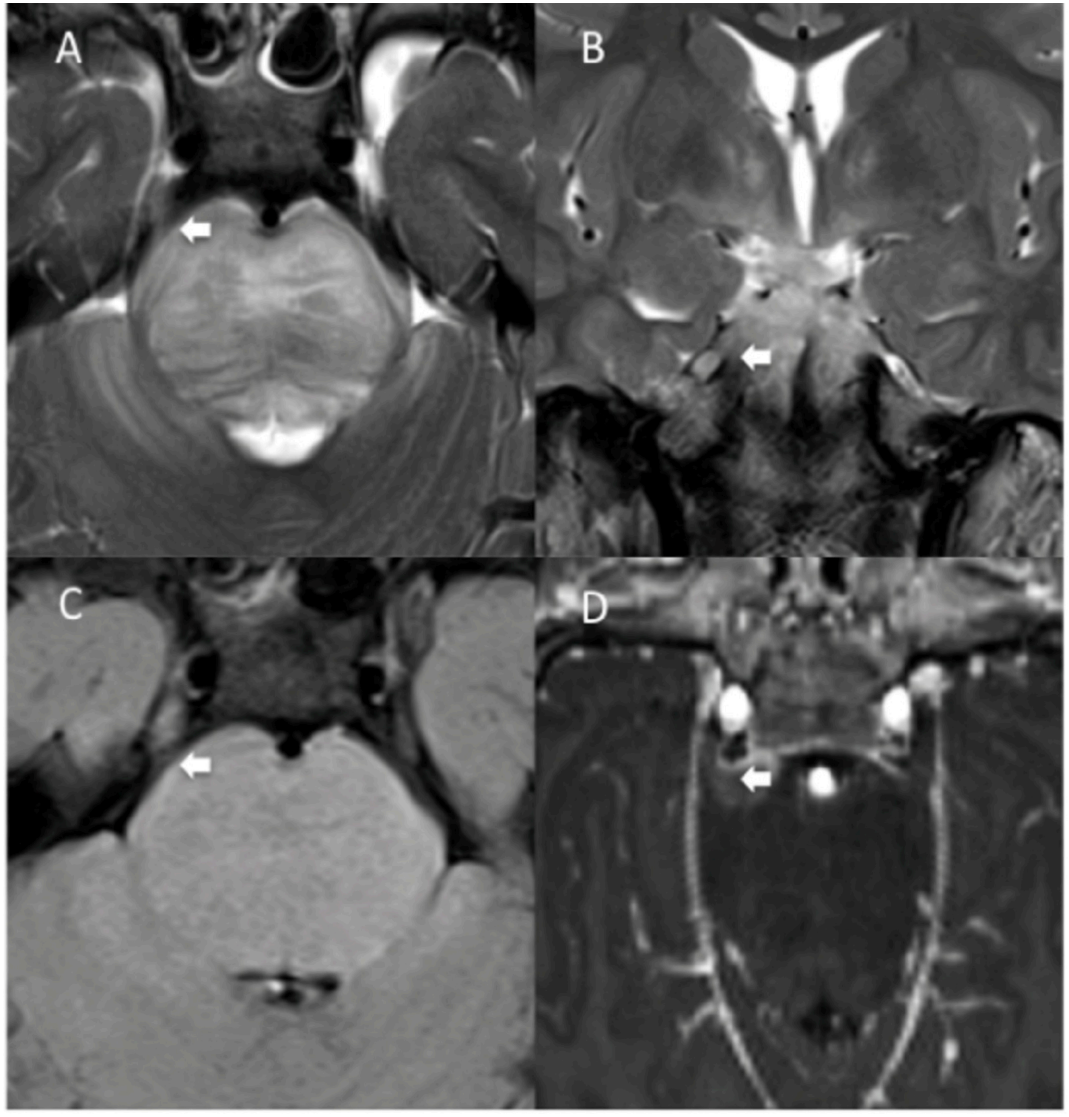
Another MRI diagnosis of direct involvement of CN V by DIPG in a 7-years-old boy. TSE T2 axial (A) and coronal (B), axial FLAIR (C), and T1 post-contrast para-axial (D) reformatted MRI images show thickening and abnormal signal intensity involving the root entry zone and the cisternal course of the right V cranial nerve, contiguous with the tumor, (A–C, white arrows). Findings are consistent with direct right V cranial nerve involvement by the tumor. There is associated contrast enhancement (D, white arrow).
